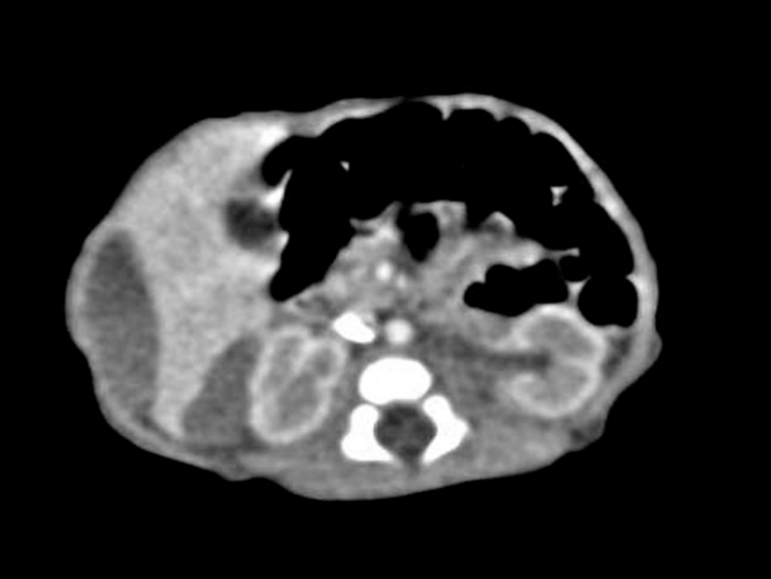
A contrast-enhanced abdominal CT scan shows a well-defined, oval shape, non-enhancing, slightly low-density cystic mass in the subcapsular area of the lateral portion of the liver and hepatorenal space.
